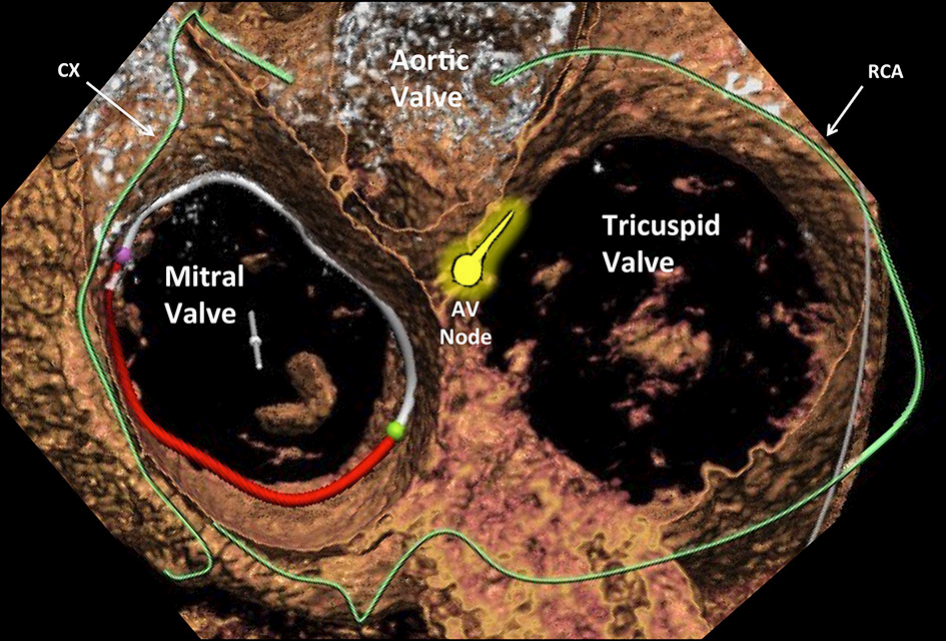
Cross-sectional view of the three heart valves seen from above with atria removed. Advanced editing of a computed tomography image performed with 3mensio (Pie Medical Imaging, Netherlands) software. The proximity of the atrioventricular valves (different area can be appreciated) to the coronary arteries that are located in the atrioventricular groove (the circumflex for the mitral and the right coronary artery for the tricuspid) represents a similar aspect to be taken into account. Cx, circumflex artery; AVN, atrioventricular node; RCA, right coronary artery.
